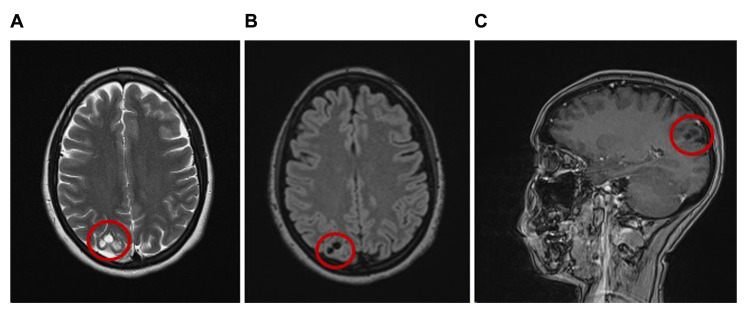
MRI brain with and without contrast of MVNT patient presented in this case report MRI brain with and without contrast at initial presentation revealed the lesion identified in the subcortical white matter in the right parietal lobe with areas of more solid T2-FLAIR hyperintensity between the cystic processes, consistent with MVNT. The axial T2 (A), axial T2-FLAIR (B), and sagittal T1 post-contrast (C) images are shown. MVNT, multinodular and vacuolating neuronal tumor; FLAIR, fluid-attenuated inversion recovery.
